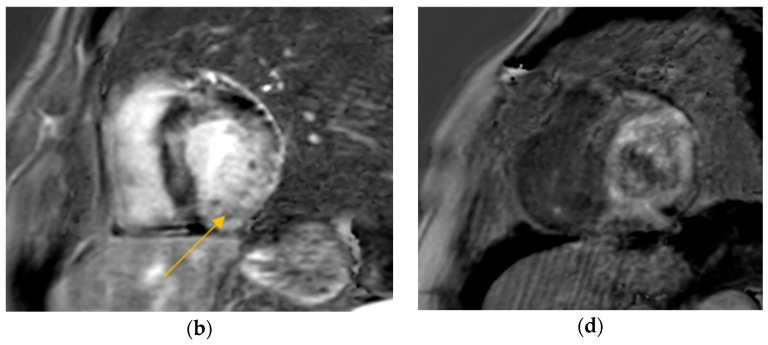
Cardiac MRI examples of late gadolinium enhancement (LGE). (a) Four-chamber view showing septal LGE (scar). (b) Short-axis view demonstrating a large inferolateral scar. Both (a,b) demonstrate the patchy LGE pattern characteristic of cardiac sarcoidosis, marked by the yellow arrow. (c) Four-chamber view and (d) short-axis view showing diffuse subendocardial and transmural LGE in a global distribution, consistent with cardiac amyloidosis.
